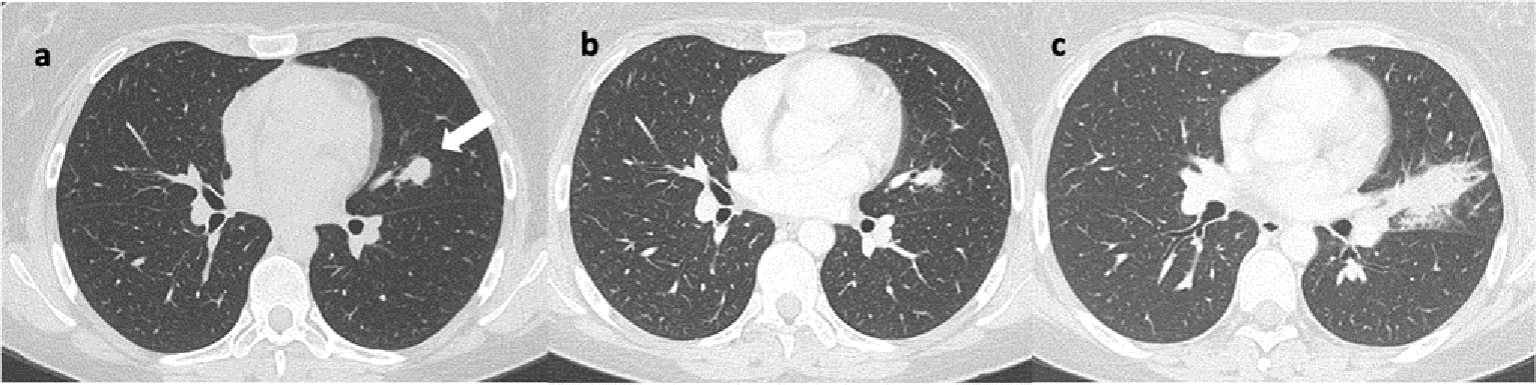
Axial lung CT scans reveal: (A) a nodular lesion in the upper lingular segment, indicated by the arrow. Image (B) shows a reduction in size at the 14-month follow-up after initiation of immunotherapy. Image (C) shows a significant increase in size at the 6-month follow-up after discontinuation of immunotherapy, characterized by spiculated margins.
